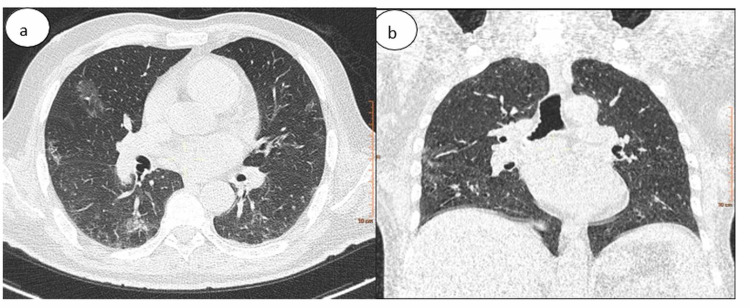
Chest CT in (a) axial and (b) coronal lung windows showing bilateral subpleural ground-glass opacities The percentage of lung involvement is approximately 25%.
